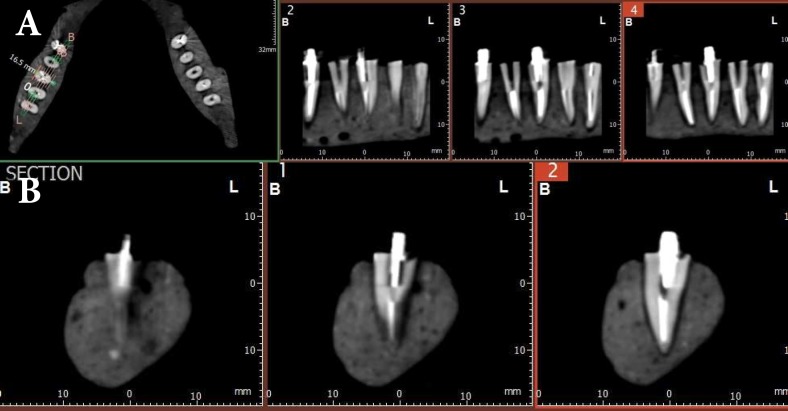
A, B) A fracture line on the right third tooth from the end of dental arch in mesiodistal cross-sectional CBCT images after applying metal artifact reduction (MAR)
